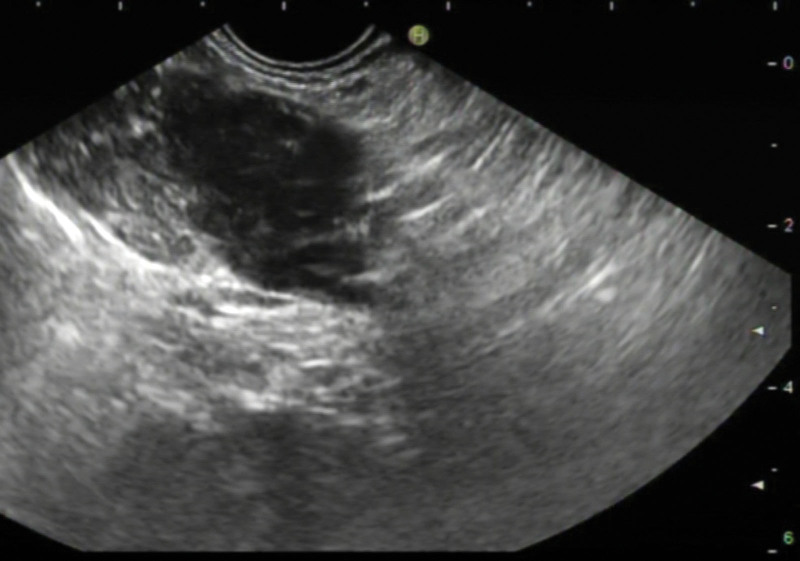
Endoscopic ultrasound (EUS) image. EUS reveals a well-defined, heterogeneous, and hypoechoic mass measuring 32 × 23 mm in the pancreatic tail. The overlying mucosal layers appear intact.
